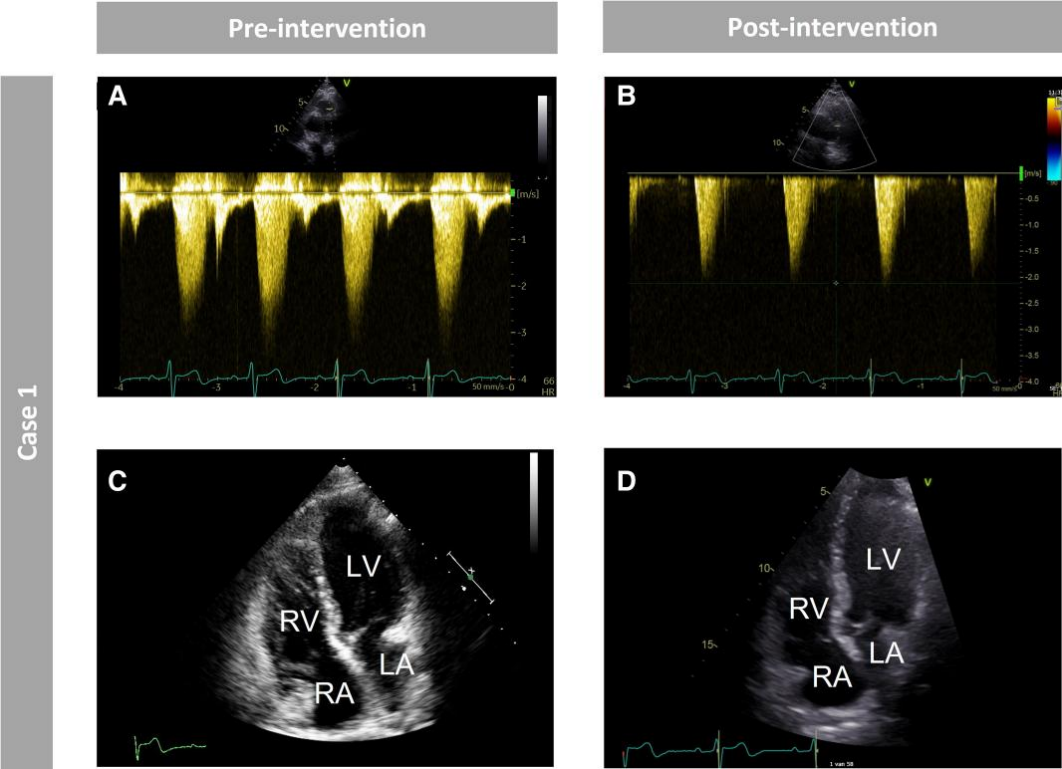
Pre- and post-interventional imaging of Case 1. *Figure 3* shows the pre- and post-interventional imaging of Case 1 using transthoracic echocardiography. (*A*) Pre-interventional peak gradient of the left pulmonary artery (LPA) measured using continuous wave Doppler echocardiography. (*B*) Post-interventional peak gradient of the LPA measured using continuous wave Doppler echocardiography. Note the different scales in the vertical axis in panels *A* and *B*. (*C*) Pre-interventional four-chamber cardiac magnetic resonance cine image. (*D*) Post-interventional RV focused apical four-chamber echocardiography image. *A*–*B* show a decrease in post-interventional LPA peak gradient compared with pre-interventional LPA peak gradient using continuous wave Doppler echocardiography. *C*–*D* show pre- and post-interventional preserved RV volumes. Right ventricular systolic function was found to be preserved during echocardiography.
